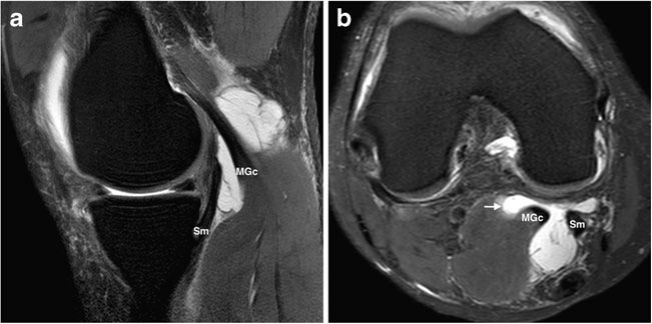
a, b. Baker’s cyst in a 33-year-old man presenting with nonspecific intermittent knee pain. Sagittal FS PD-weighted MRI (a) shows a hyperintense multiloculated fluid collection surrounding the medial gastrocnemius tendon. Its typical emergence between the medial head of the gastrocnemius muscle and the semimembranosus tendon is more evident on the axial view (b), as well as an intramuscular extension in its lateral aspect (arrow). MGc, medial gastrocnemius; Sm, semimembranosus
